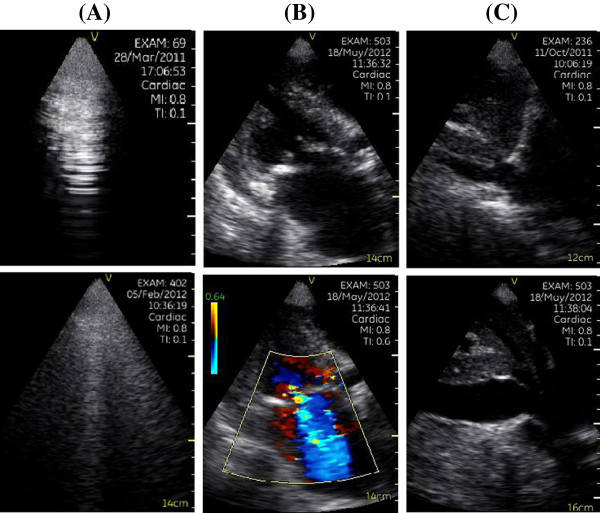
Imaging of lung-cardiac-inferior vena cava(LCI)integrated ultrasound. (A) Imaging of the lung ultrasound: Alines (upper) and B-lines (lower). (B) Imaging of the cardiac ultrasound: Apical long-axis view (upper) and moderate mitral regurgitation (lower). (C) Imaging of the inferior vena cava ultrasound: Collapsibility ≥ 50% (upper) and < 50% (lower).
